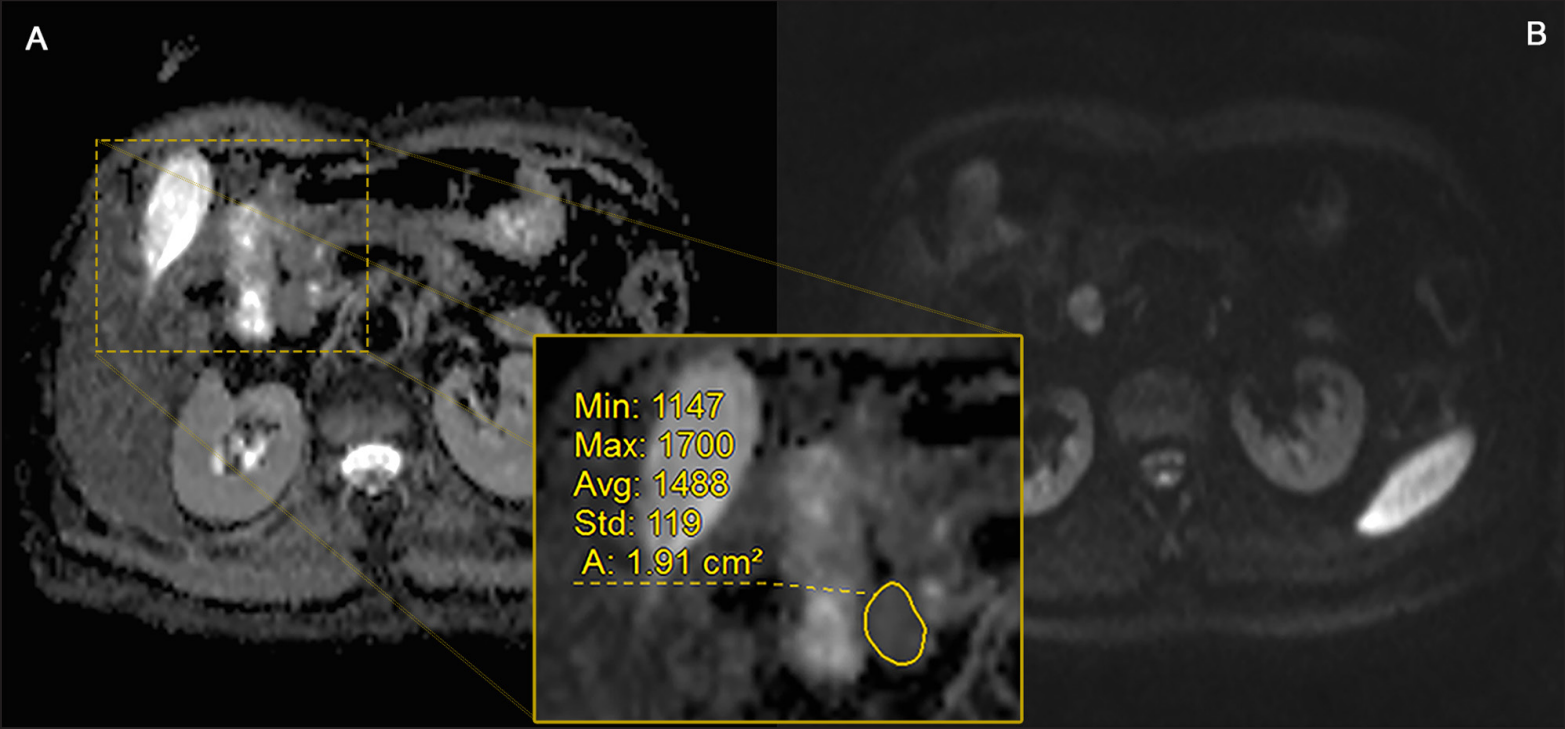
ROI placement (grade 1). Axial ADC map (A) and DWI b1000 (B) image with ROI placement along the borders of a WHO G1 lesion (resection proven paraduodenal metastasis) in a 52-year-old female.
